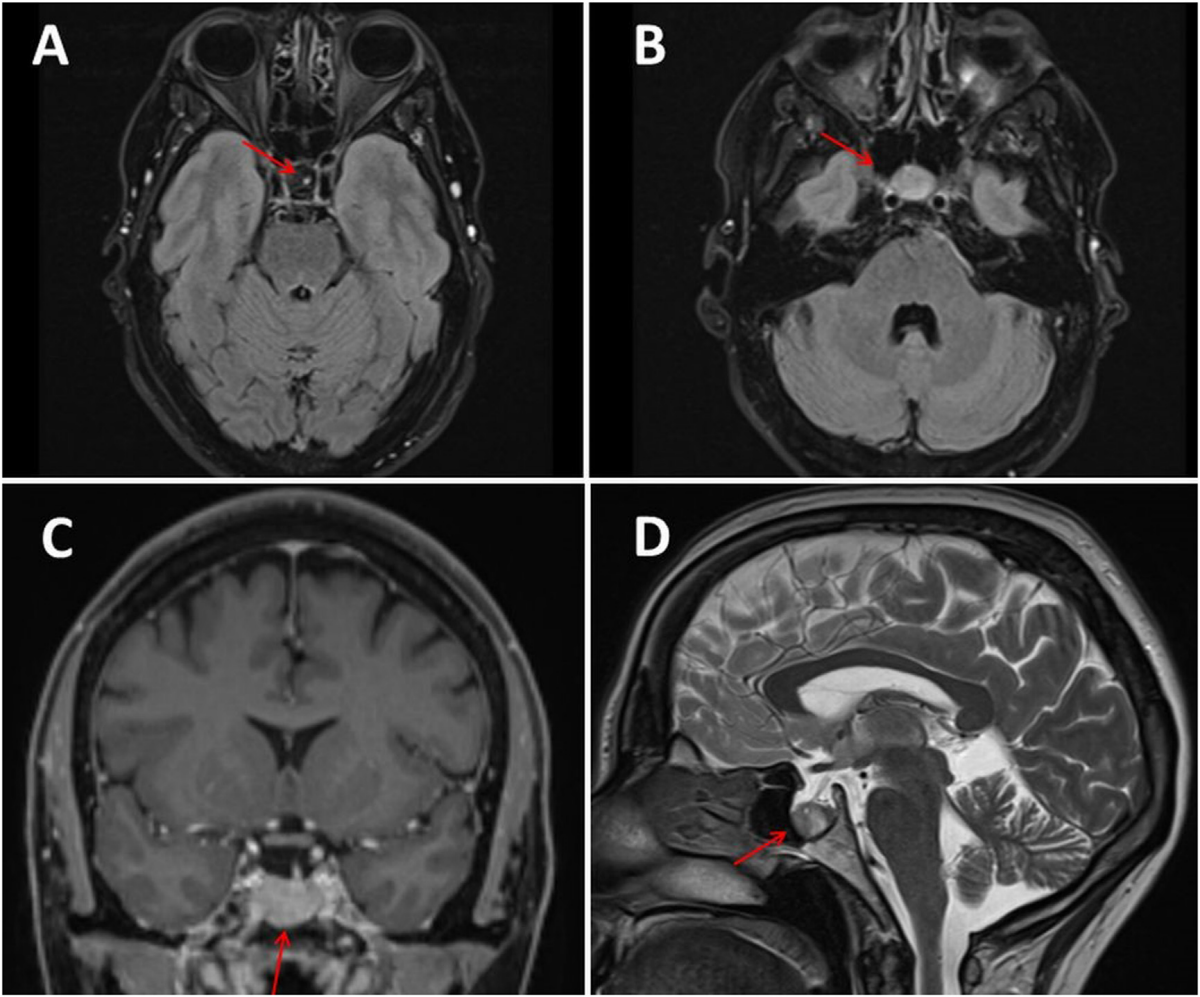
Cerebral MRI with contrast medium showing a mass lesion within the spheroid sinus and prominent pituitary stalk (panel A‒D), suggesting macradenoma of the anterior lobe of the pituitary gland.
